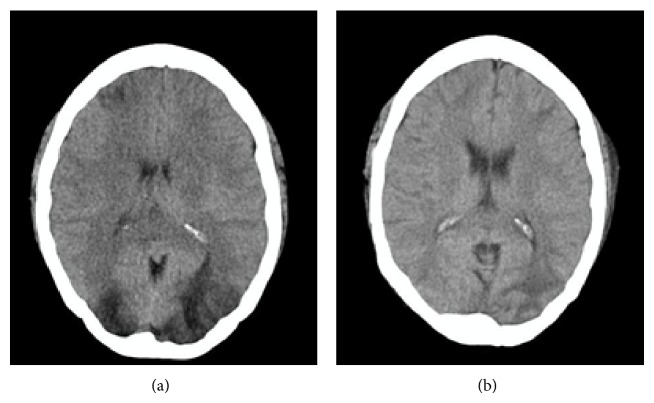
(a) Brain CT scan showing hypodense lesions in subcortical white matter of frontal and occipital lobes. (b) Brain CT 8 days after initiation of neurological symptoms, showing significant reduction of previously described hypodensities.
